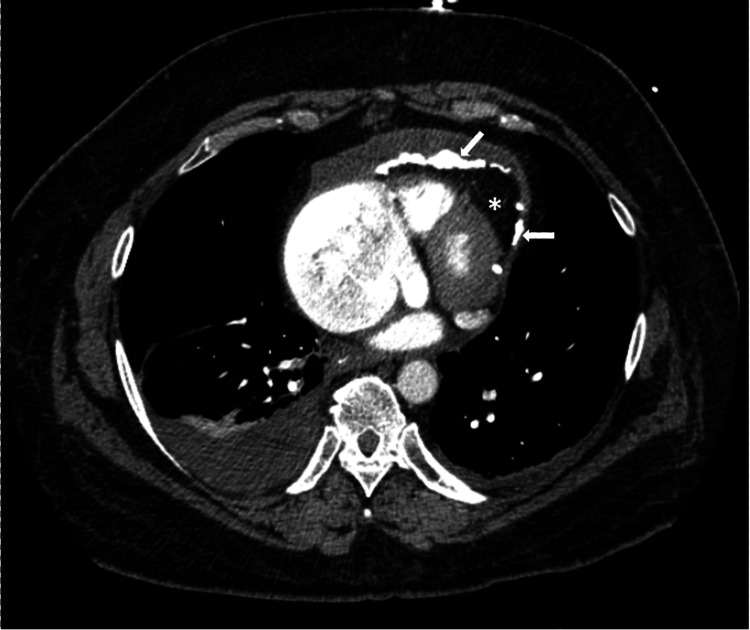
Chest computed tomography Chest computed tomography demonstrating pericardial effusion (asterisk) and pericardial calcifications (arrows).
